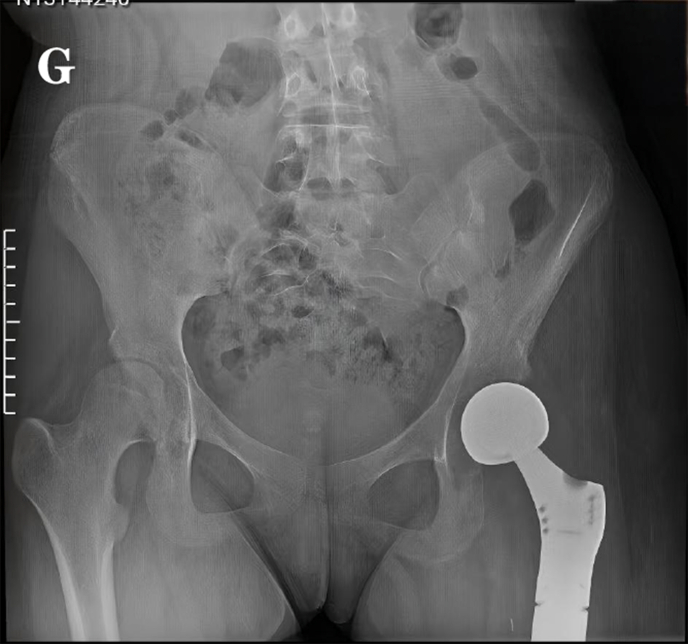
The X-ray image two months after the limb-sparing surgery.
